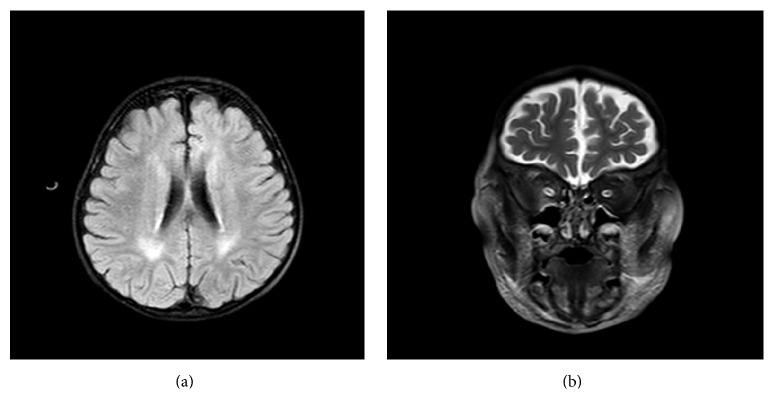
Brain magnetic resonance images: (a) The axial FLAIR image shows periventricular white matter signal changes compatible with hypomyelination. (b) Coronal T2-weighted magnetic resonance image shows bilateral optic atrophy and cerebral atrophy.
